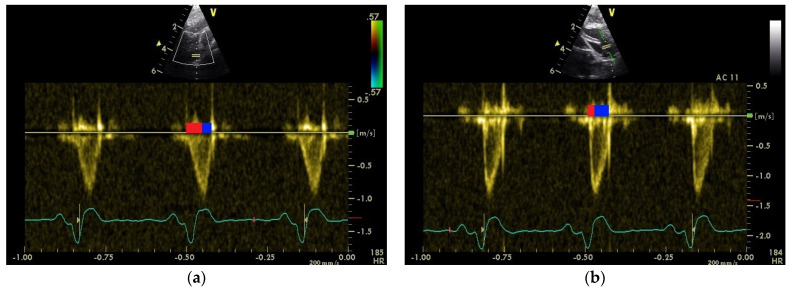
PW Doppler sonographic blood flow profile of the pulmonary artery (a) and the aorta (b). The acceleration phase of the blood flow is marked with a red line, and the deceleration phase with a blue line.
